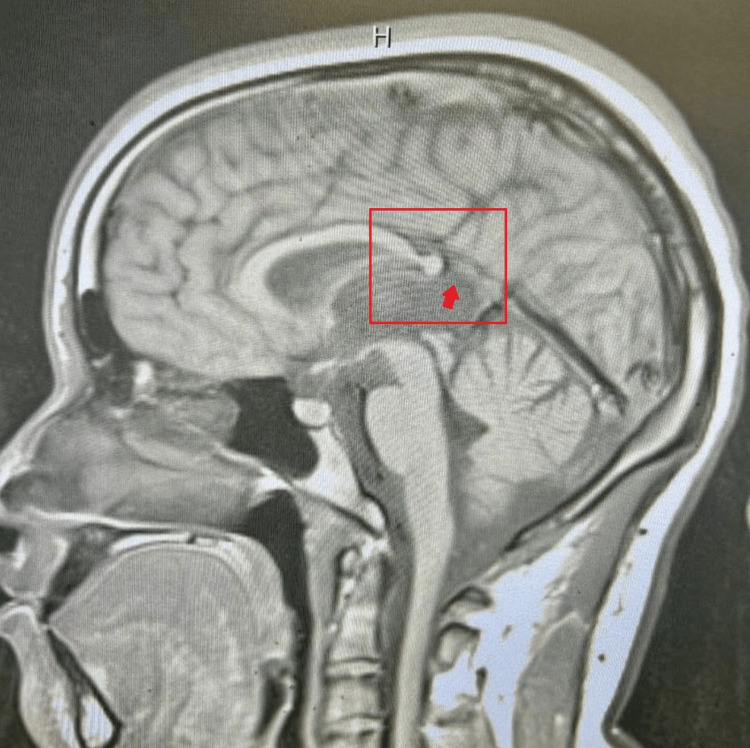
Sagittal T1-weighted MRI. Mid-sagittal T1-weighted MRI demonstrating truncation of the posterior corpus callosum. The splenium is absent (red rectangle), while the rostrum, genu, and body are preserved. The arrow indicates the expected location of the splenium, which is absent.
